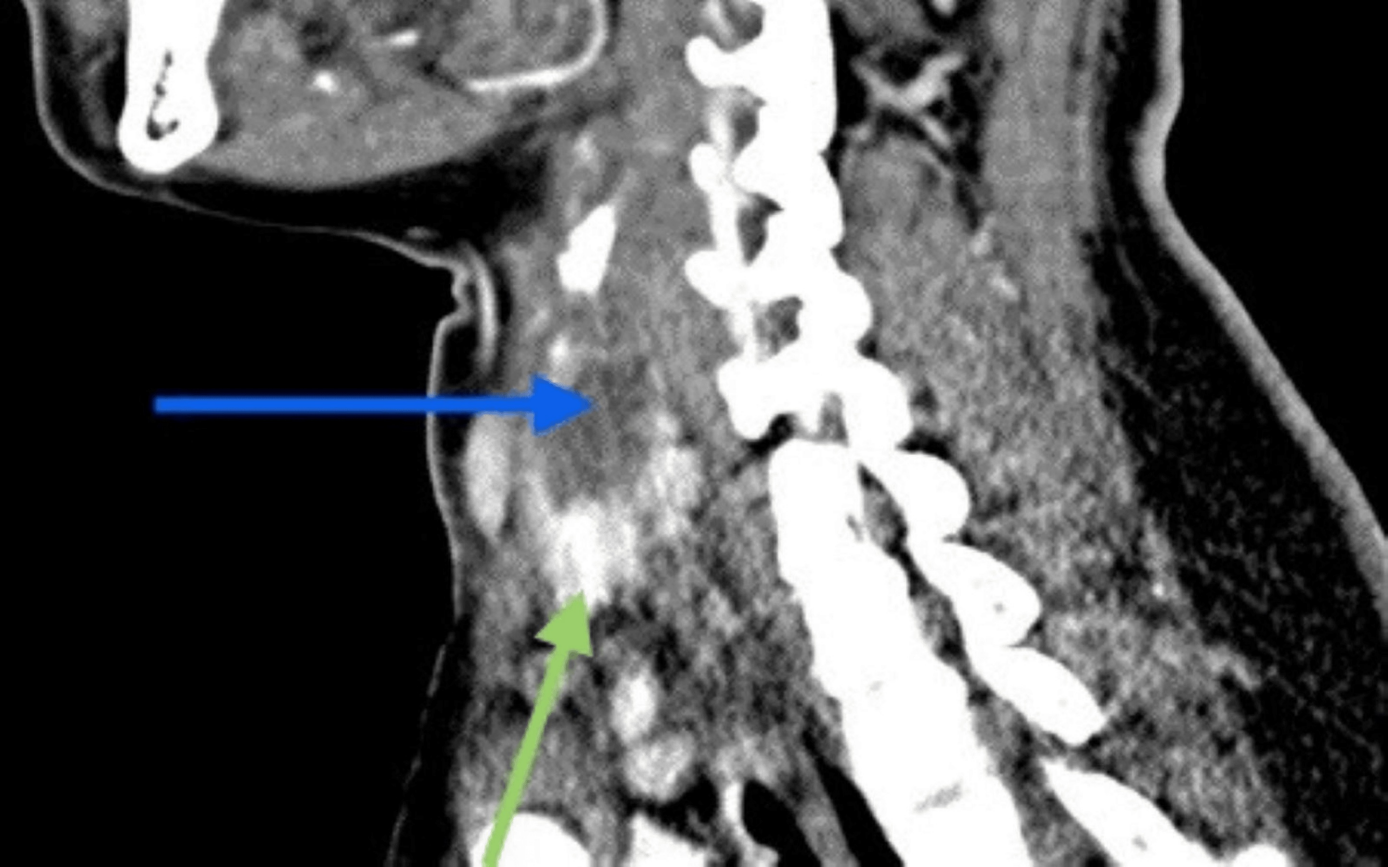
CT sagittal view shows circumferential thin fluid seen surrounding the trachea (blue arrow), adjacent to the thyroid gland (green arrow).
